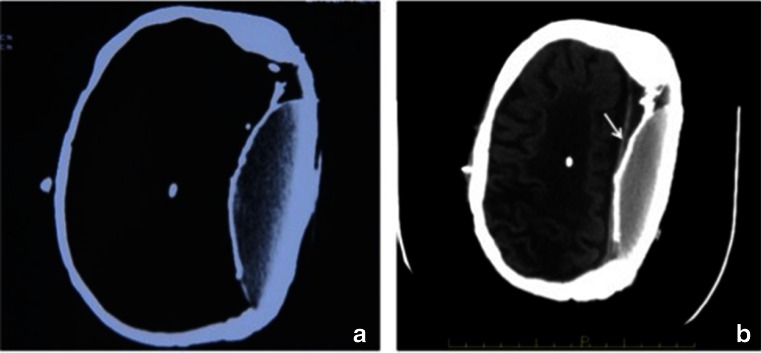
Axial CT from 1996 (a) and 2013 (b). Both CT show left hemispherectomy and filling-reduction cranioplasty. The left cavity is filled by a breast silicone implant covered by a metallic mesh. Medially to the implant, there is a calcified lyodura. Right V-P shunt is seen. The appearance on the 2013 CT (b) is unchanged comparing from the 1996 (a)
